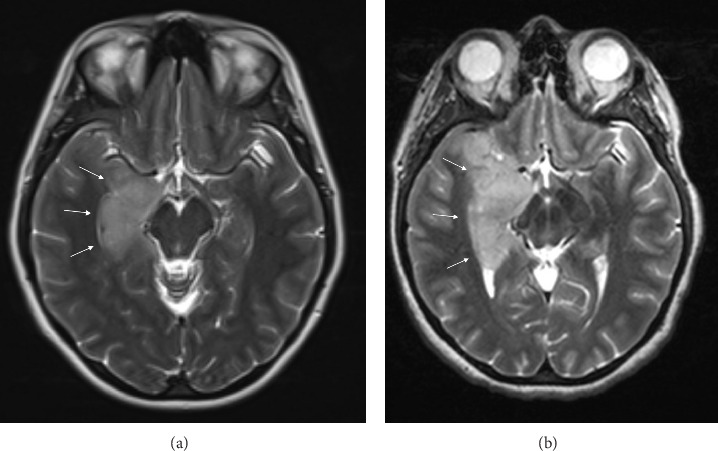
(a) Initial MRI head, T2-weighted sequence, demonstrating an area of hyperintensity (denoted by arrows) in the right mesial temporal lobe measuring 2.5 × 2.6 × 3.1 cm, and (b) 3-month follow up MRI head, T2-weighted sequence, demonstrating interval progression of mesial temporal lobe mass (again, denoted by arrows), now measuring 3.5 × 3.3 × 5.5 cm.
